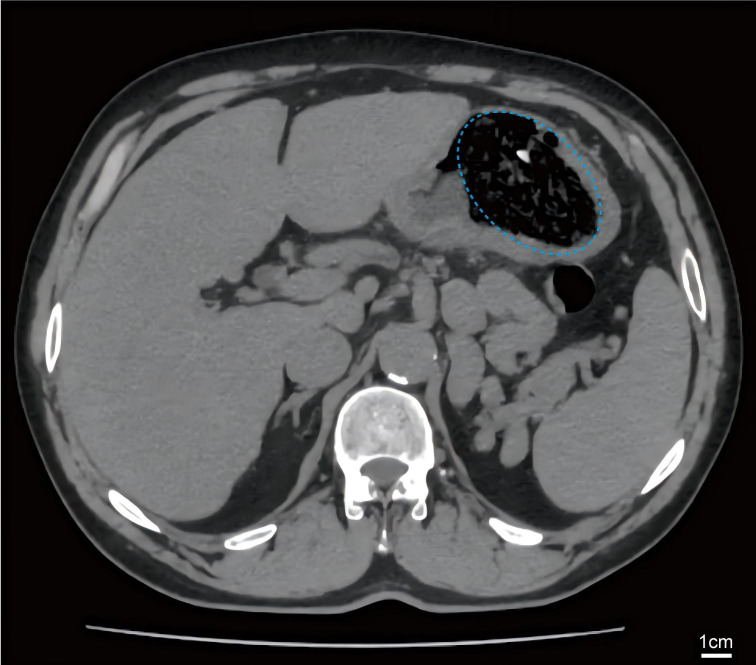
Computed tomography revealed a giant gastric bezoar.
